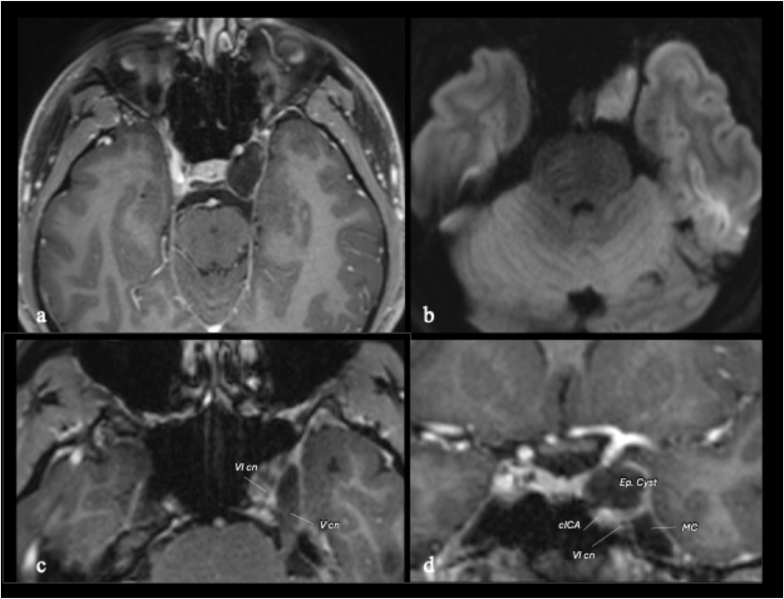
Preoperative axial MRI demonstrating a lesion located in the superolateral compartment of the cavernous sinus, hypointense on T1-weighted images and without contrast enhancement (a), associated with marked diffusion restriction on diffusion-weighted imaging (DWI) (b), findings highly suggestive of an epidermoid cyst. (c, d) Axial and coronal T1-weighted contrast-enhanced images showing inferolateral displacement of the abducens nerve (VI cn) with respect to the cavernous internal carotid artery (cICA). Meckel's cave (MC) is not invaded by the epidermoid cyst.
